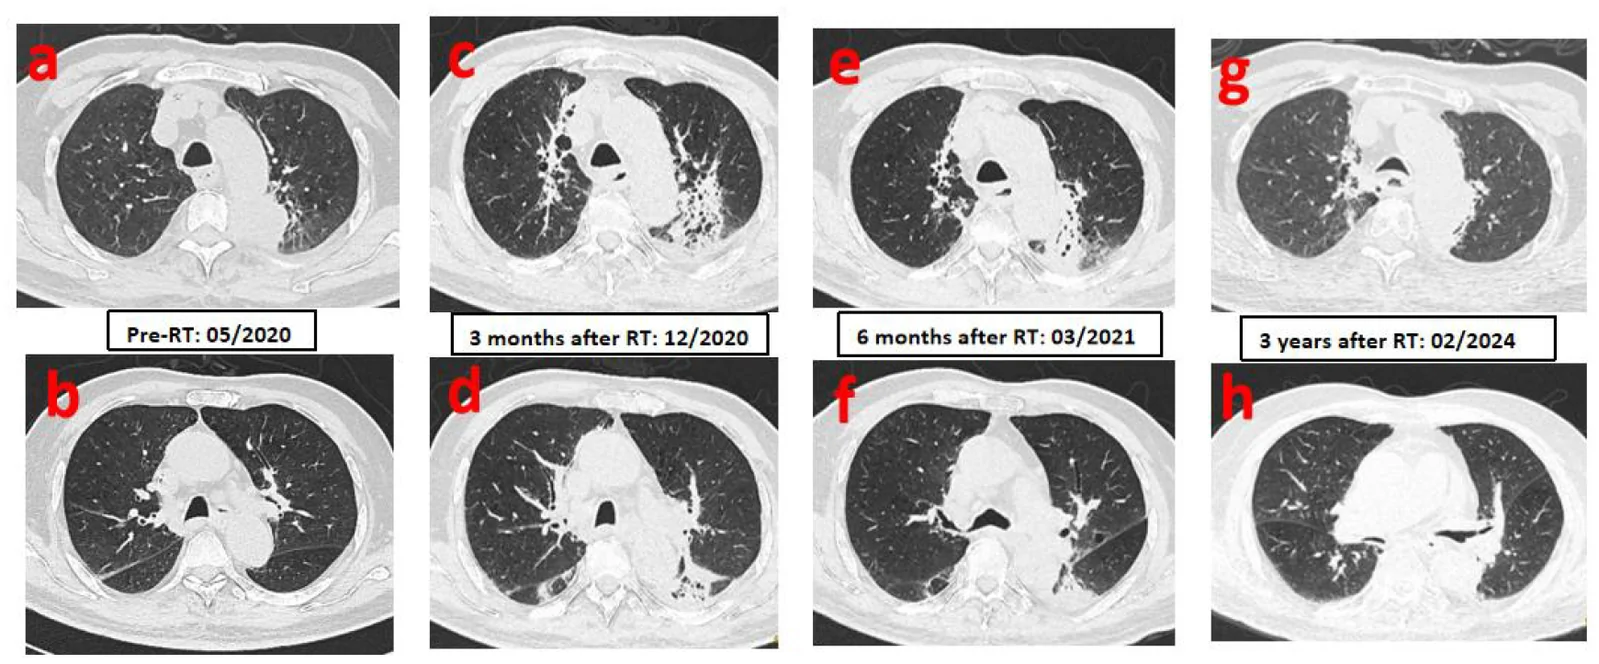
Dynamic changes in lung parenchyma after radiotherapy. Follow-up CT scans were shown after radiotherapy: Chest CT images at the initial visit (a, b); left upper lobe radiation pneumonitis at 3 months after radiotherapy (c, d); radiation-induced lung fibrosis at 6 months after radiotherapy (e, f); and chronic fibrotic changes in the left lung tumor area more than 3 years after radiotherapy (g, h).
